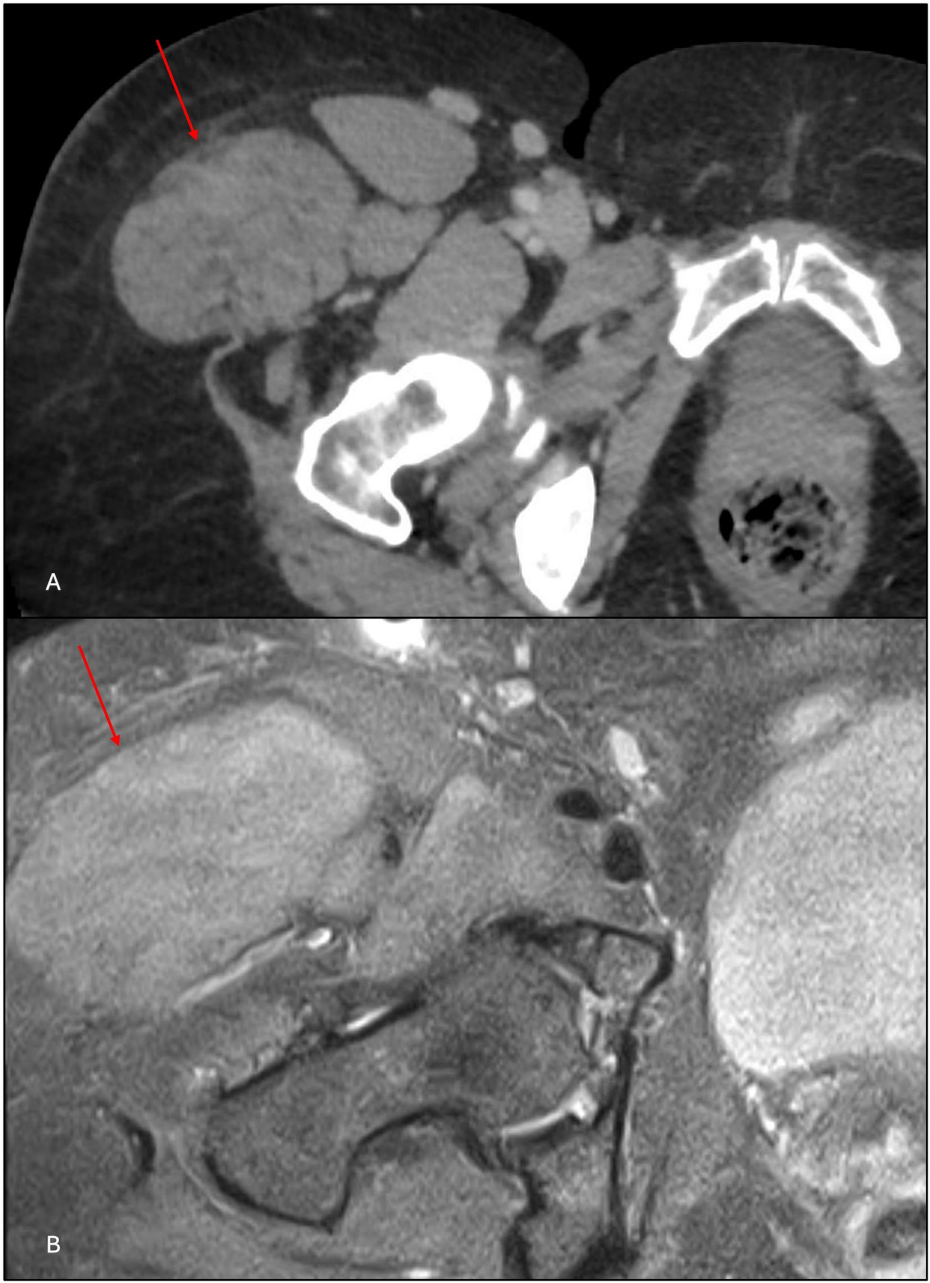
(A) Contrast-enhanced CT from January 2025 and (B) T1 fat-saturated MR images from October 2021 show right inguinal lymphadenopathy and enlargement of the right tensor fascia lata that are stable over 4 years.
